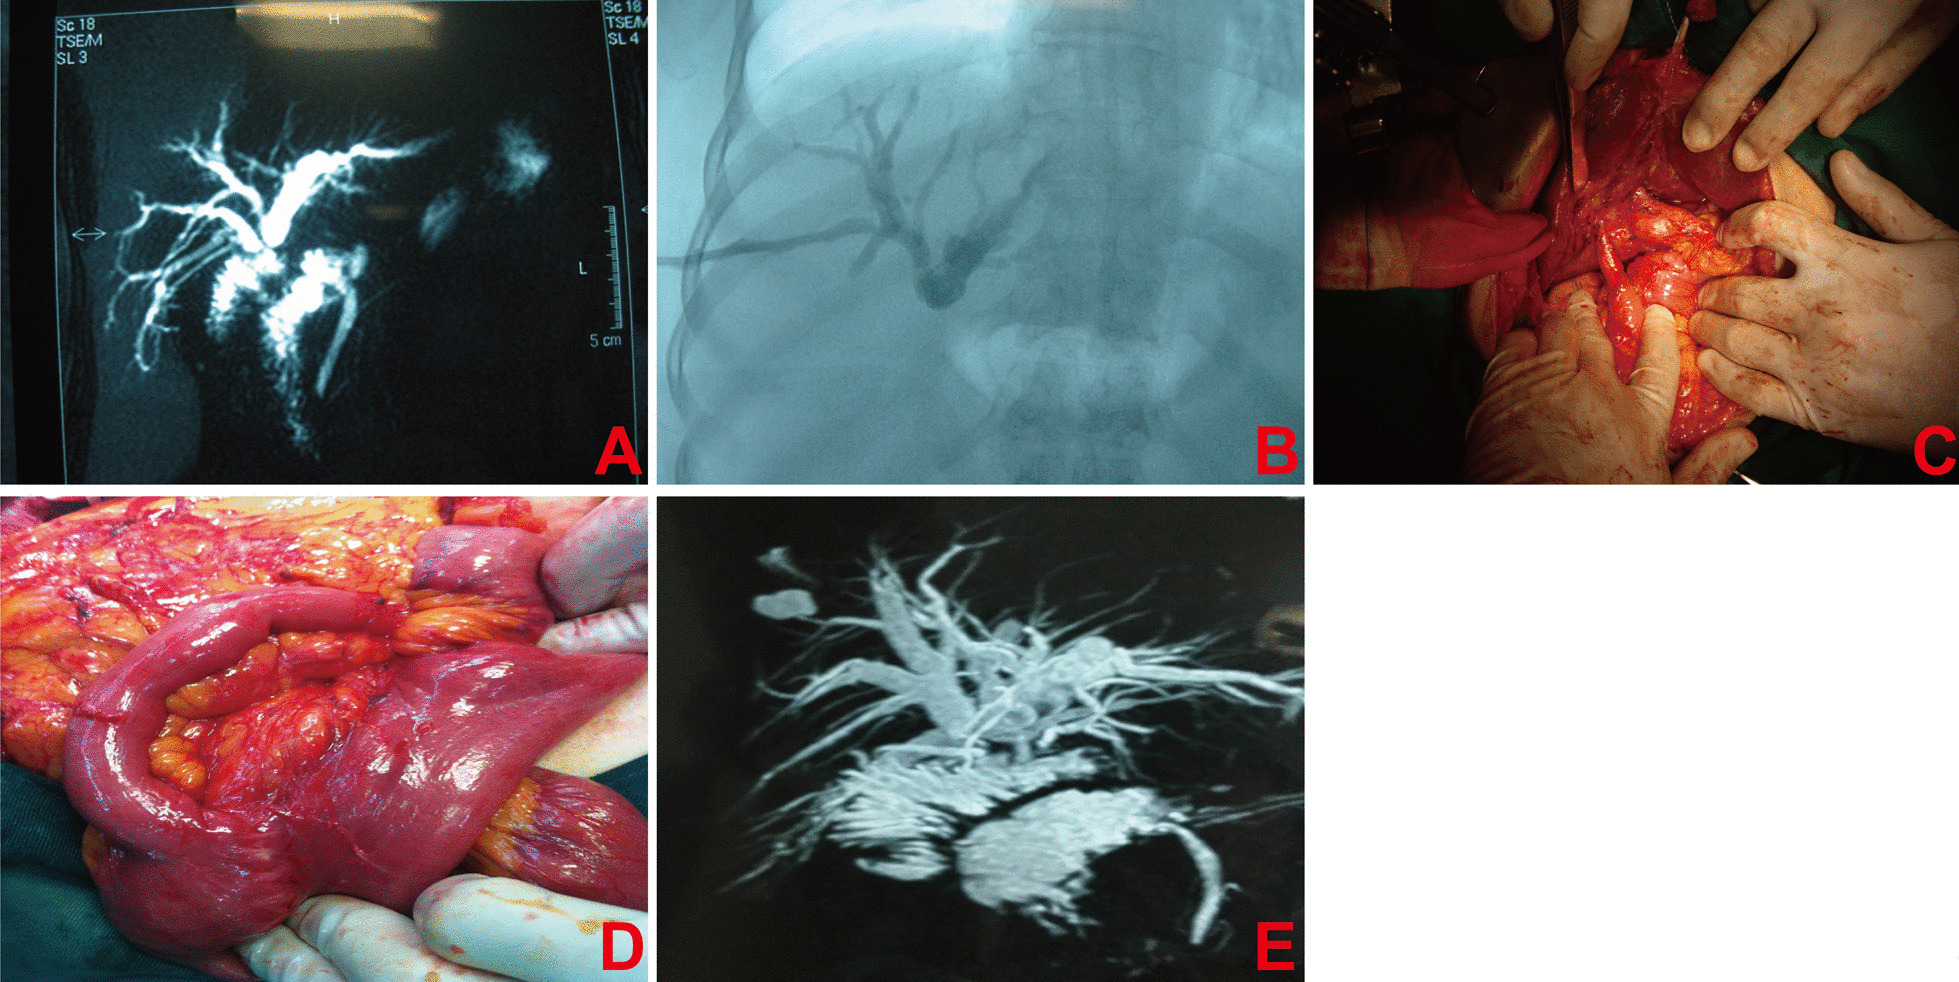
Typical imaging and intraoperative photos of the patients with traumatic biliary stricture. A Preoperative transhepatic cholangiography showed that there formed a common trunk between the right anterior bile duct confluence and the left hepatic duct, and there was obvious biliary stricture in the left hepatic biliary orifice. B Radiography indicated that there was no anastomotic stoma, suggesting that the common trunk between the right anterior bile duct and the left hepatic duct was not anatomized. C Intraoperative imaging demonstrated obstruction of duct-jejunum anastomotic stoma and shortening of intestine in the biliary output loop. D Intraoperative imaging showed the significant difference between biliary output loop and the normal intestine. E Postoperative transhepatic cholangiography indicated obstruction of anastomotic stoma and intrahepatic biliary duct calculosis after cholangiojejunostomy
